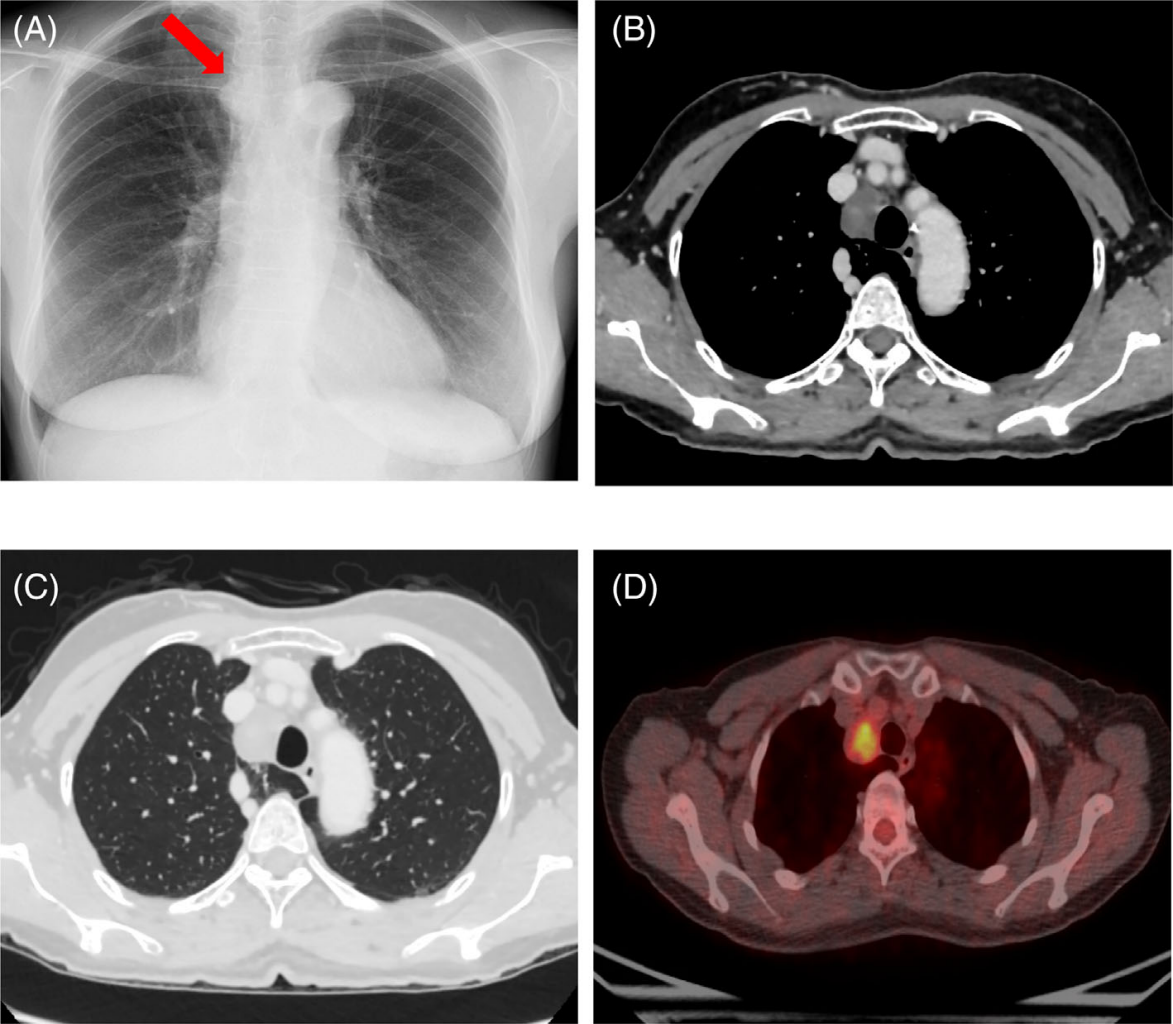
(A) Chest X‐ray revealed a mass in the upper mediastinum. (B) contrast‐enhanced CT revealed a 3‐cm solid lesion with a smooth surface and poor contrast effect slightly excluding the right wall of the trachea, but there was no tendency to infiltrate the surrounding tissue. (C) This case had an azygos lobe near the tumour. (D) ^18^F‐fluorodeoxyglucose positron emission tomography (FDG‐PET) showed a slight uptake in the tumour
